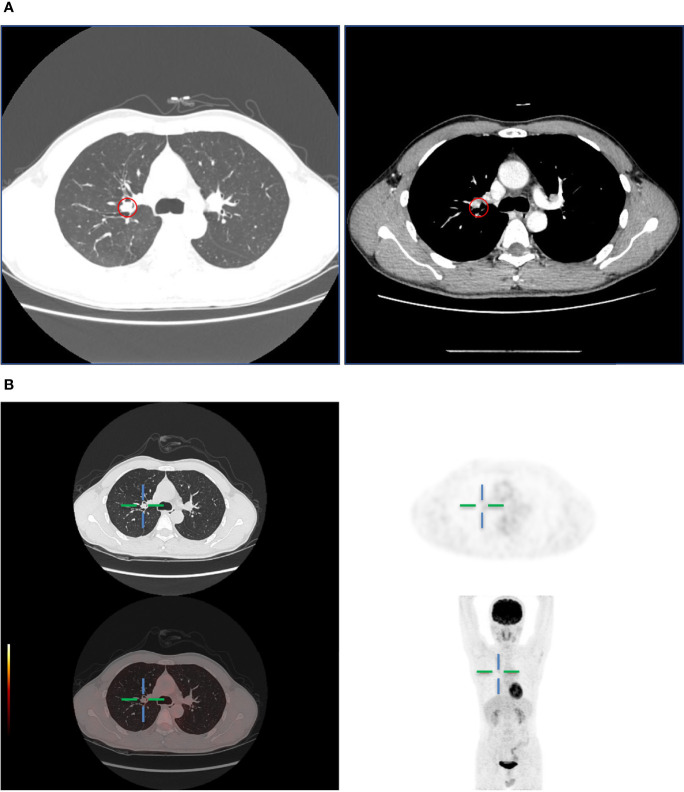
(A) Computed tomography showed a lung mass in the upper right lobe. (B) PET-CT showed mild metabolically active lung mass.
